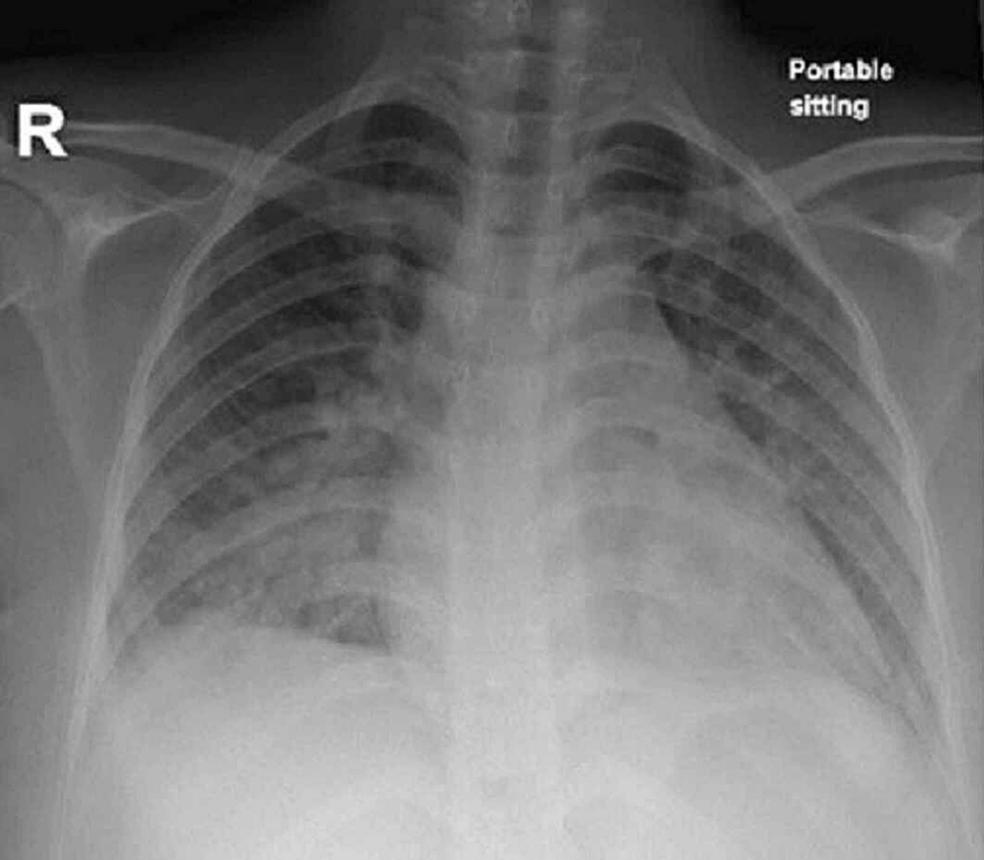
Portable anteroposterior chest X-ray showing evidence of pulmonary edema following administration of oxytocin.
